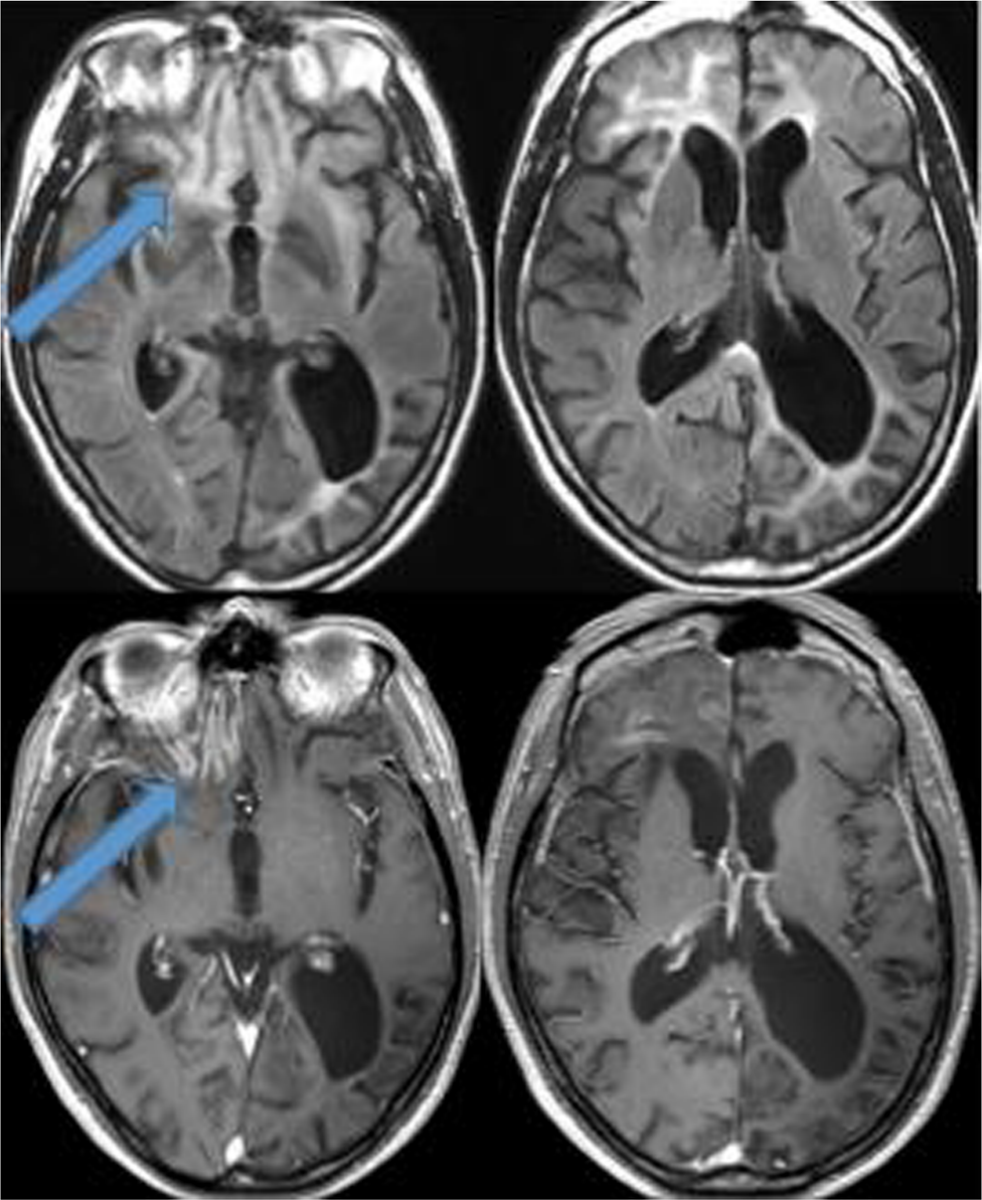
Case one, 2011: FLAIR MRI sequences from 2011 again show hyperintensity in the right basifrontal (see *arrows*) and left parieto-occipital regions, now with enhancement on corresponding post contrast T1 images (*lower row*) in the right basifrontal region only
